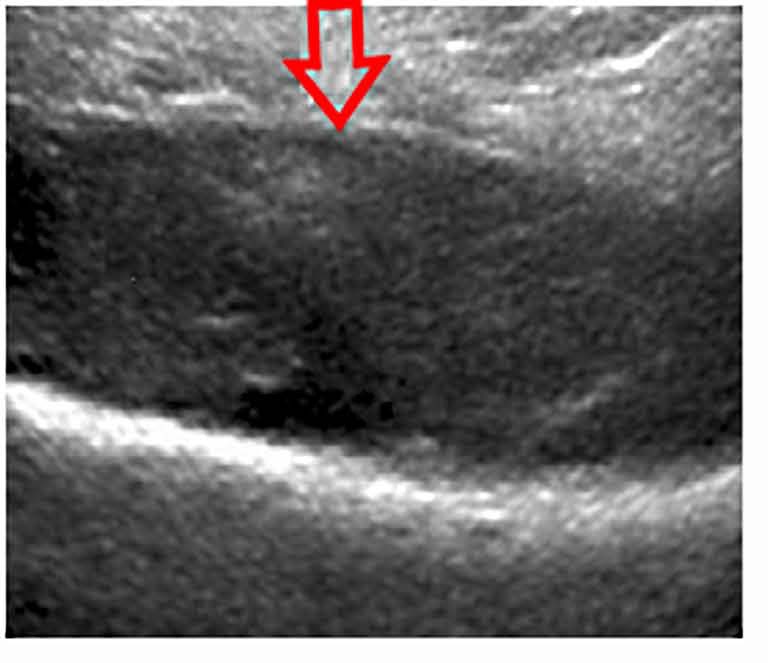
The sagittal ultrasound image shows heterogeneous hypoechoic lesions compatible with subperiosteal abscess (red arrow).
